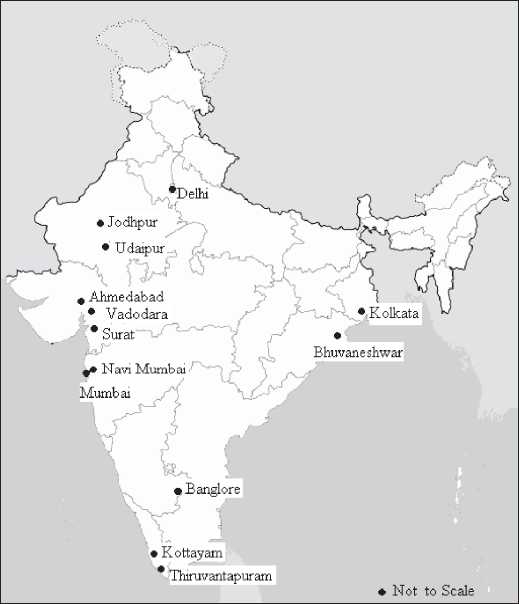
Radiological safety and QA audit carried out at various diagnostic X-ray installations in India
